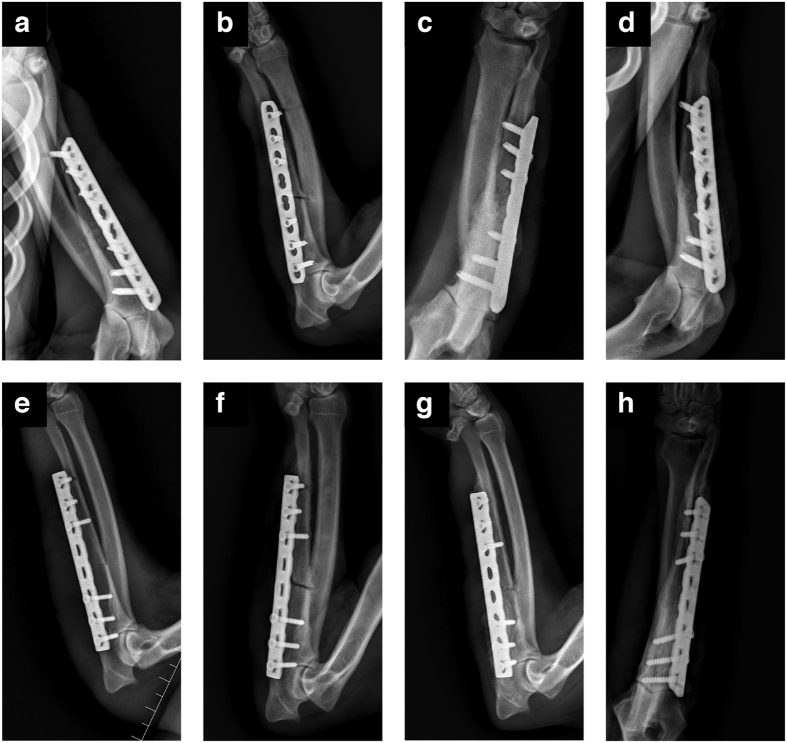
Radiographic images of fractures fixed with plates on day 1 and 4, 8, and 12 weeks after the operation. (a–d) Locking compression plate (LCP). (e–h) Dynamic compression plate (LC-DCP).
